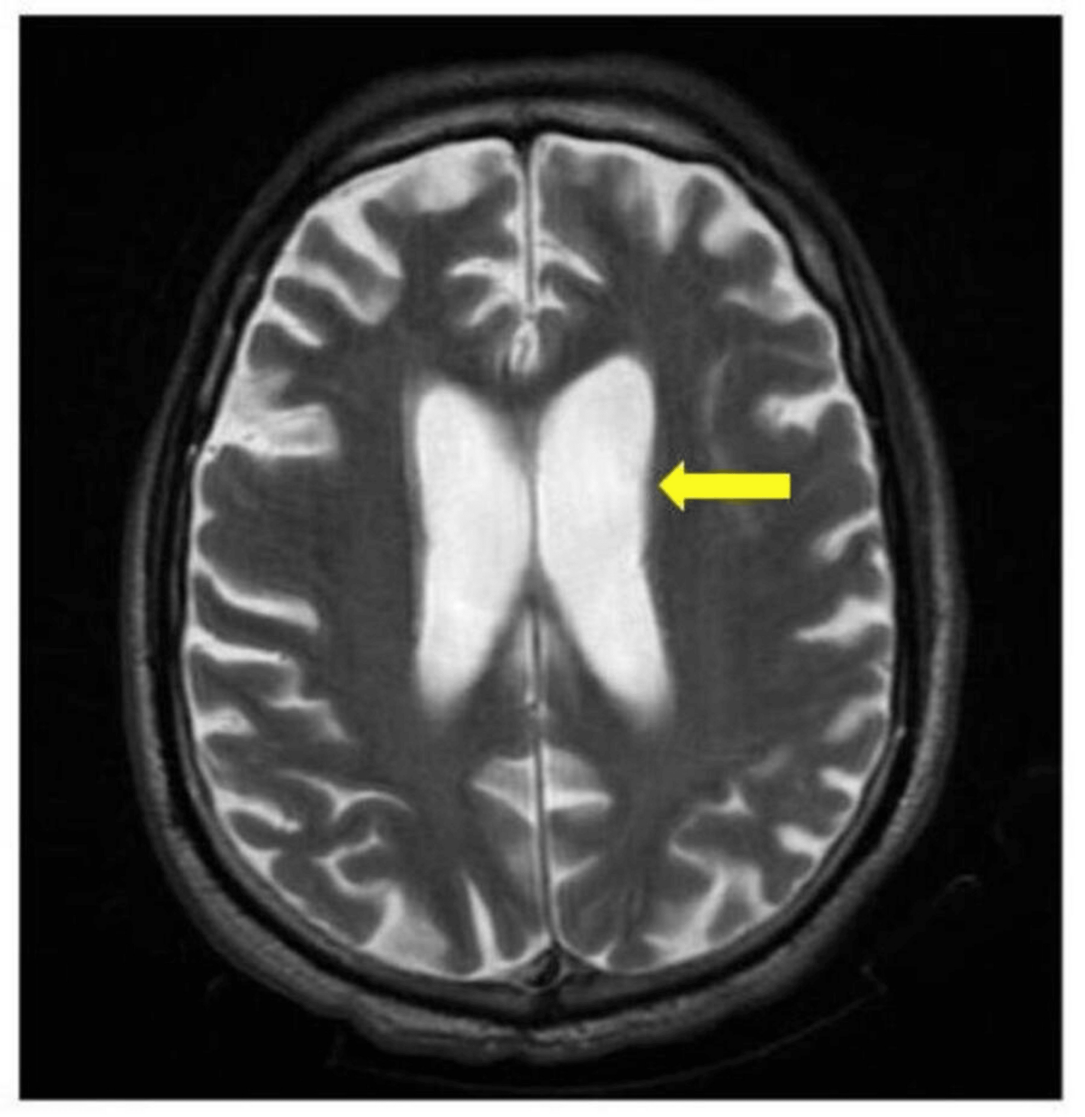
A plain MRI brain showing age-related neuroparenchymal changes with prominent sulcal spaces and ventricle
